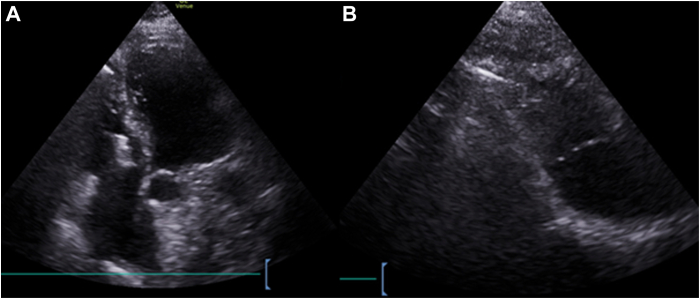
(A) Apical 4-chamber and (B) modified parasternal echocardiographic views revealing the positions of the tricuspid valve clips and the pacemaker device.
